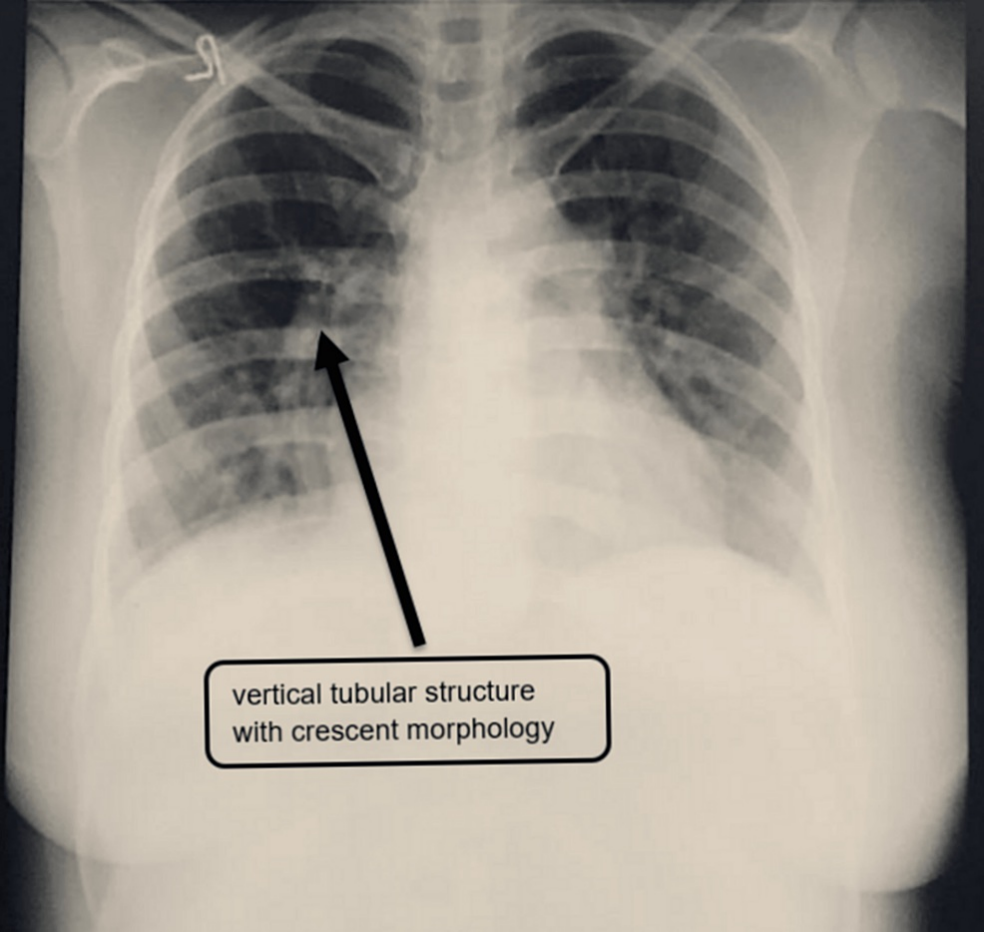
A chest X-ray shows a vertical tubular structure with crescent morphology.
